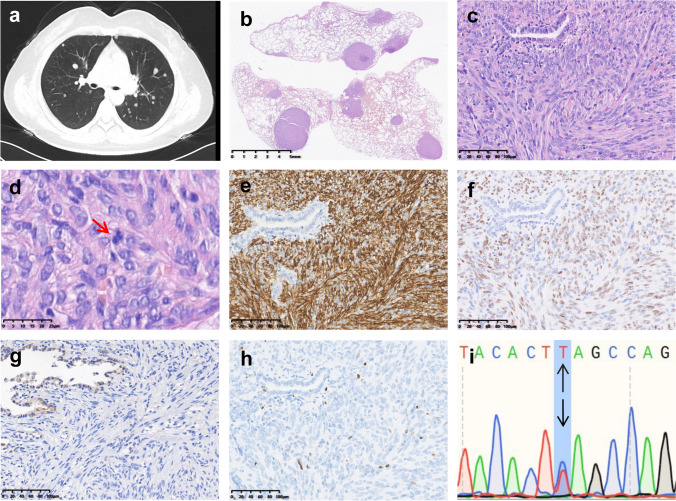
FH-d UL with lung metastasis in Case 1. a CT image showing multiple nodules with smooth, clear borders and uniform density in both lungs. b Numerous round or oval tumor nodules in the lung excision. c Entrapped native alveolar glands were observed within the tumor. d A mitotic figure is marked by a red arrow. Tumor cells were positive for e desmin and f ER and negative for g FH. h The Ki-67 index was less than 5%. i The same mutation of *FH* gene in the tumor
